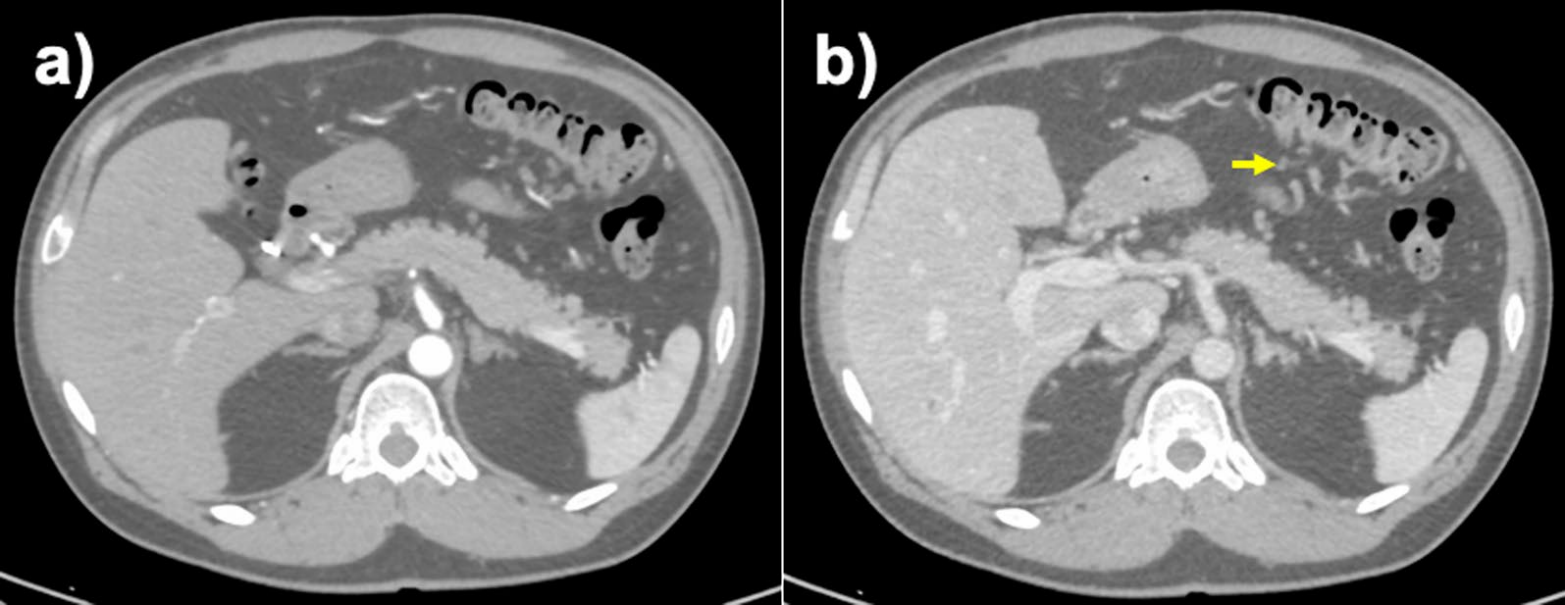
Contrast-enhanced CT examination was performed at 3 months after surgery. a In the arterial phase, transverse colonic varices (yellow arrows) were not enhanced. b In the portal venous phase, the transverse colonic varices were gradually enhanced
